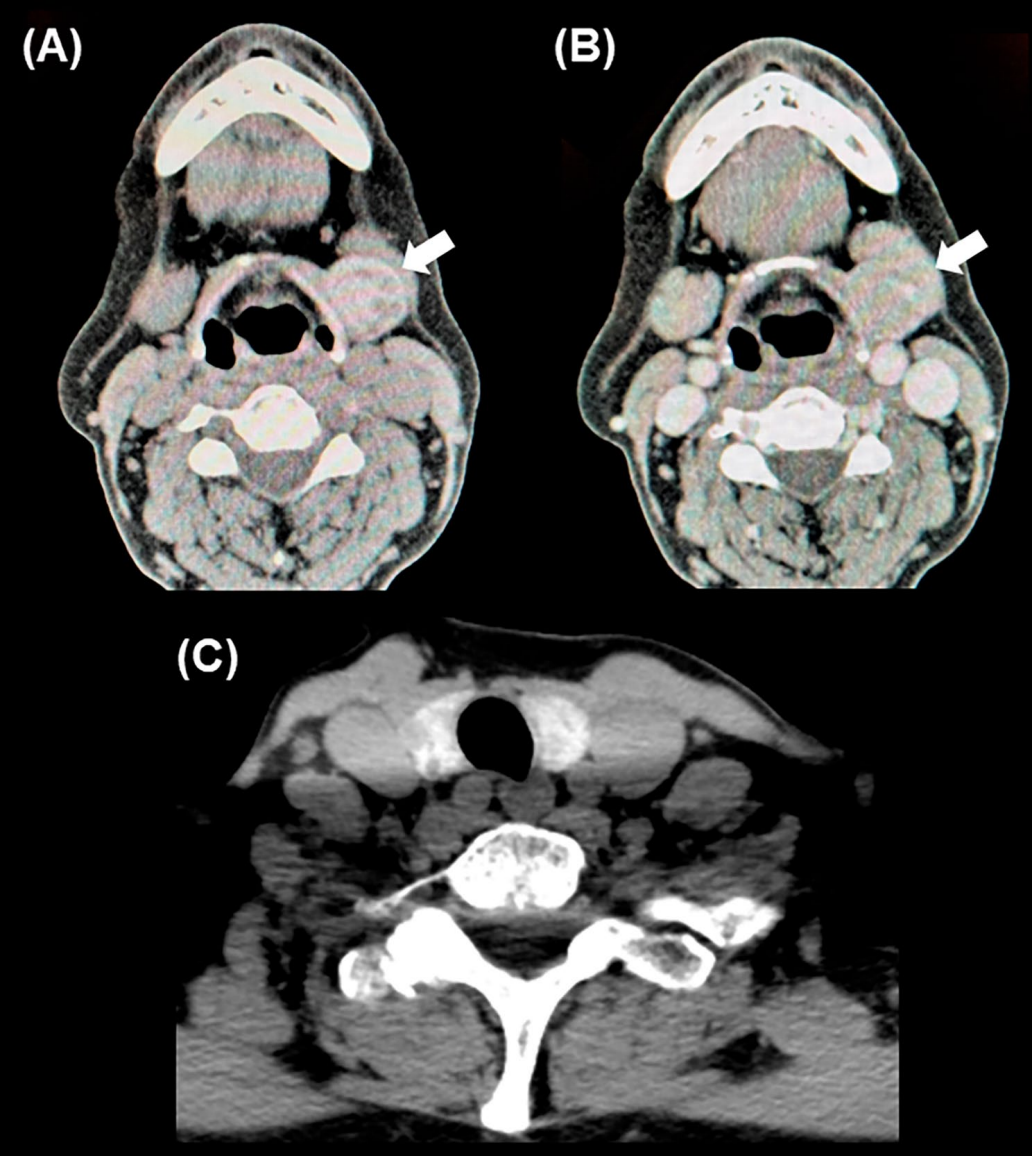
Computed tomography image of the neck. A Well-defined mass is located behind the left submandibular gland (white arrow). Without iodine contrast, the mass was mostly hyperdense and had low density. B The mass was hardly enhanced by the iodine contrast. C Thyroid gland position
